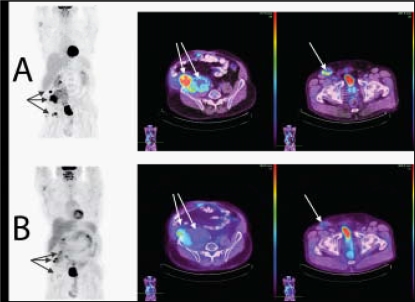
Combined positron-emission tomography and computed tomography images (A) before and (B) after third-line treatment.
